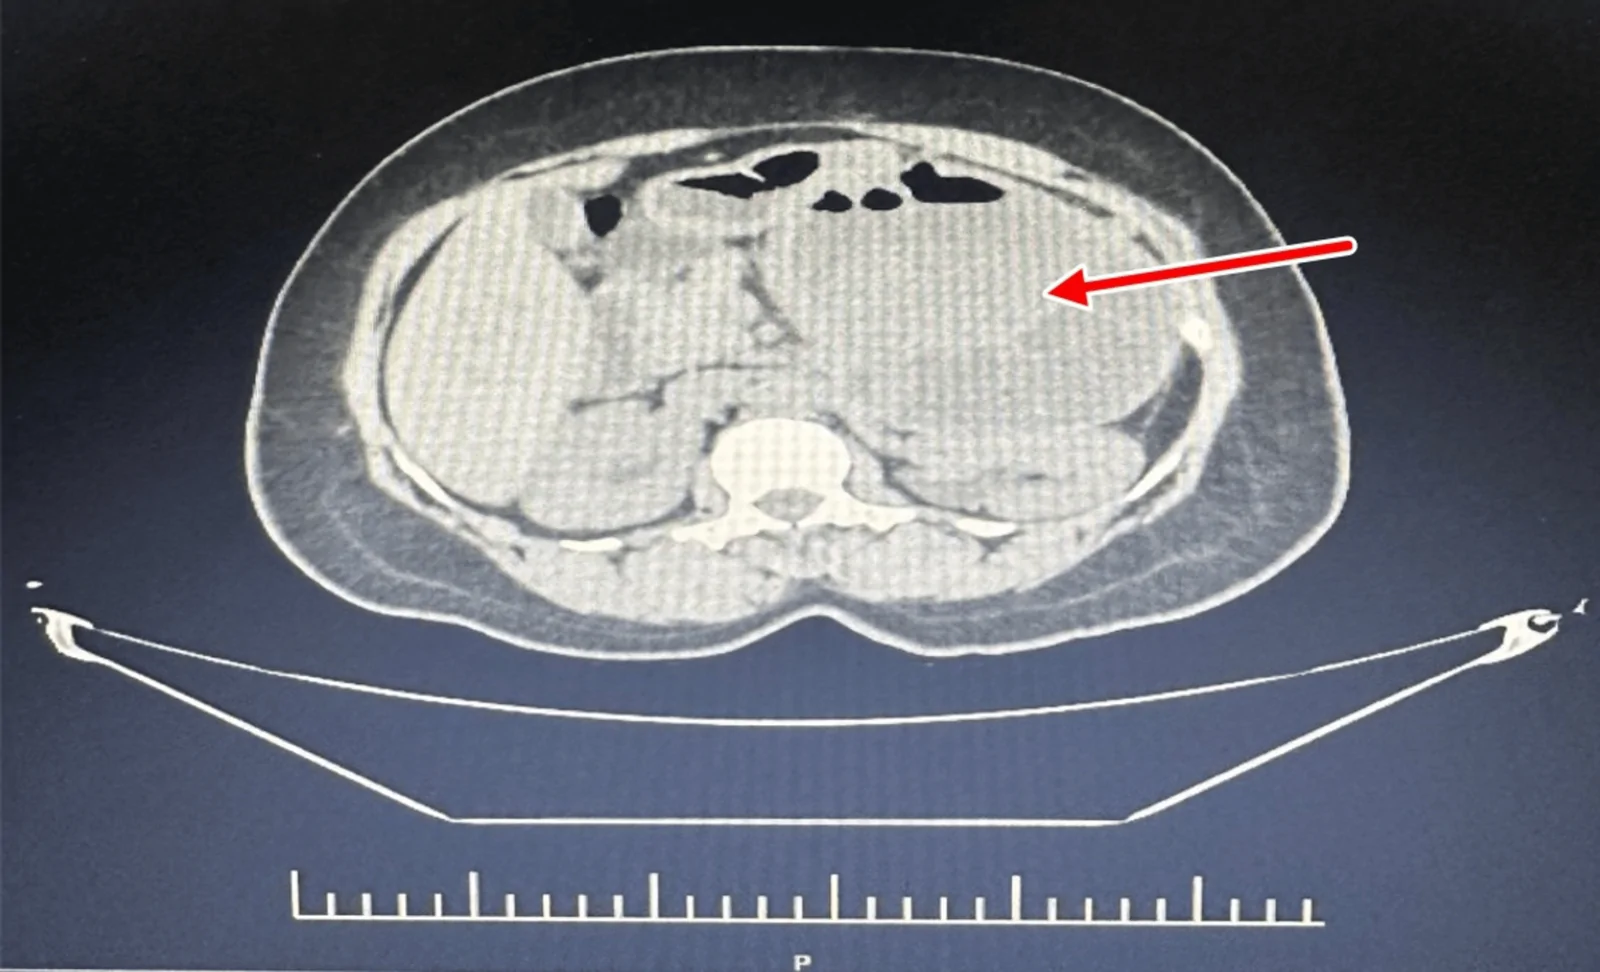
Plain CT image of the abdomen showing a large cystic lesion involving the body and tail of the pancreas (red arrow indicates the cystic pancreatic lesion)
